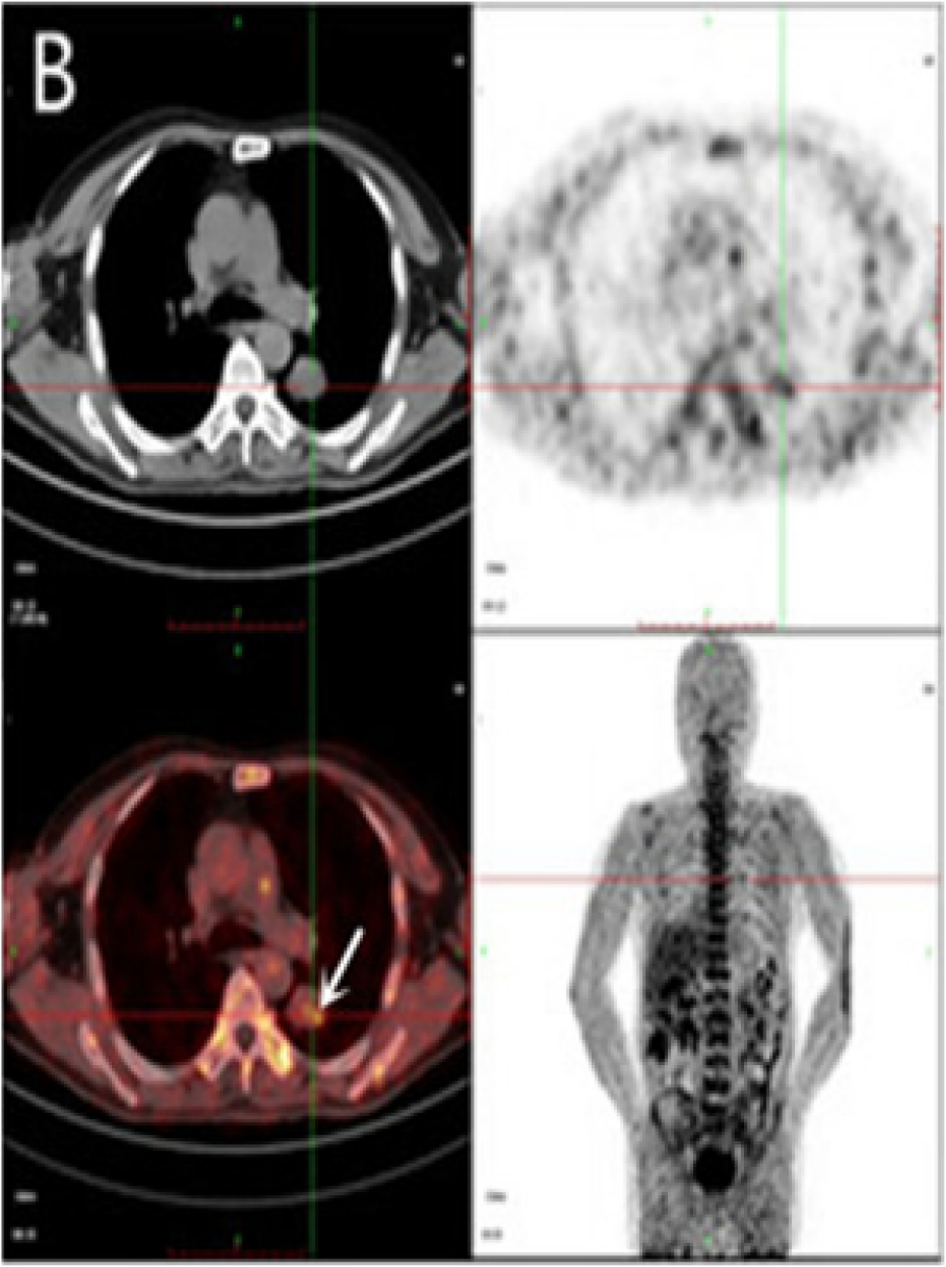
PET imaging Major organs and regions uptake at 2 h after injection of ^18^F-FMISO (B) in small cell lung cancer patient. Arrows point to tumor. (Wei Y, Zhao W, Huang Y, Yu Q, Zhu S, et al. (2016). A Comparative Study of Noninvasive Hypoxia Imaging with ^18^F-Fluoroerythronitroimidazole and ^18^F-Fluoromisonidazole PET/CT in Patients with Lung Cancer. PLOS ONE 11(6): e0157606. https://doi.org/10.1371/journal.pone.0157606).
